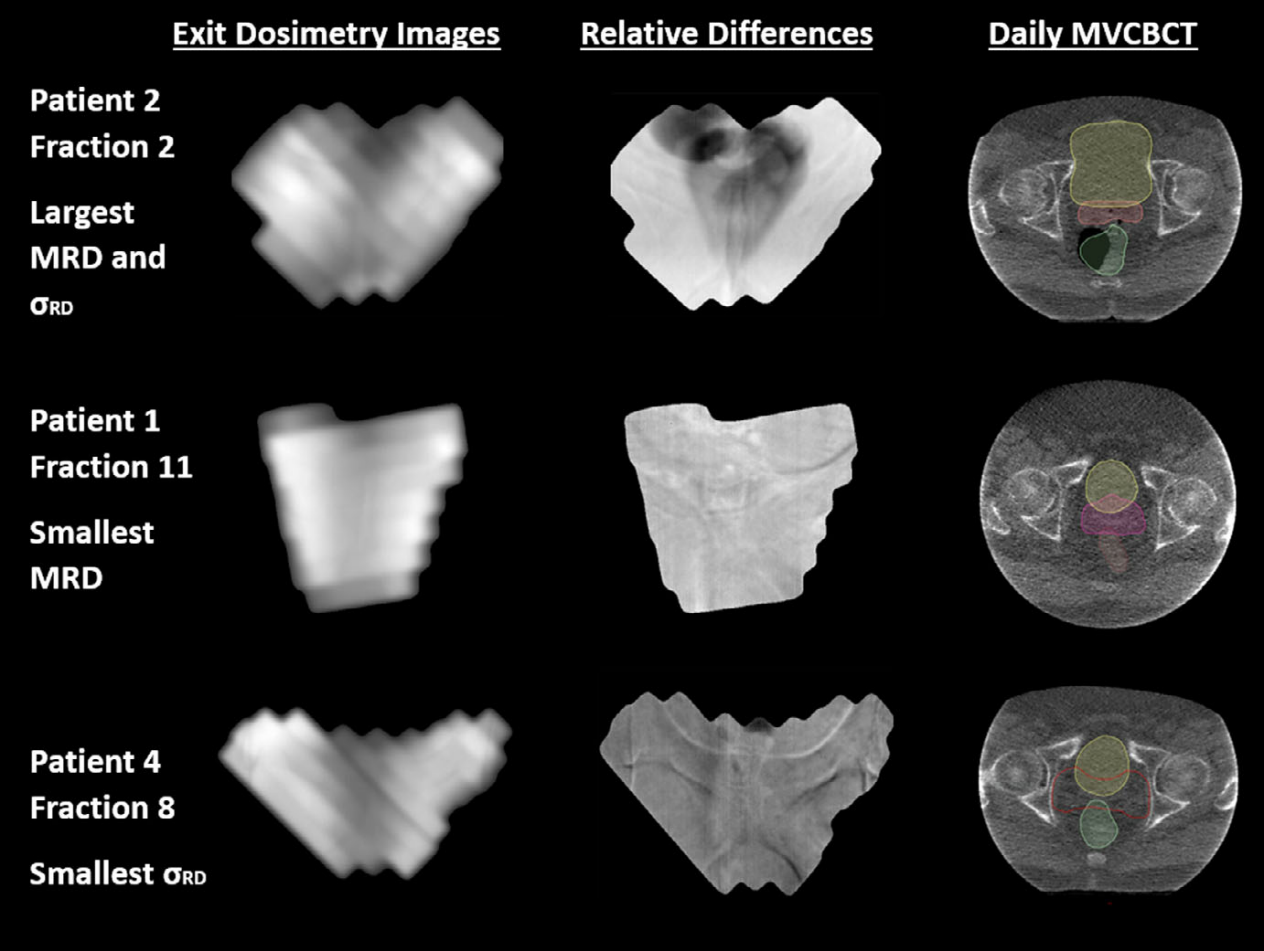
Examples of the patient exit dosimetry images from three fractions as well as the calculated relative difference between these fractions and the average image. A large gas bubble is immediately visible on the relative difference images from patient 2 fraction 2 which had the largest value for mean relative difference and *σ_RD_*. The pubic symphysis is apparent on all the calculated relative difference images. Note that the MV cone‐beam computed tomography images were acquired prior to setup correction.
